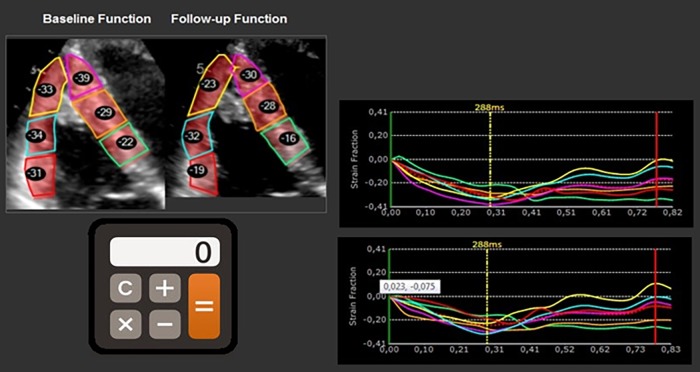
Successful tracking of a 6-segment region of interest (ROI) in a right ventricular (RV)-focused apical four-chamber view. The operator may either approve the tracing or manually exclude the septal segments by clicking on the respective segments. Then, RV free wall longitudinal strain (RVFWLS) was computed by averaging the peak systolic strain values of the three segments of the free wall (from base to apex), displayed by software.
